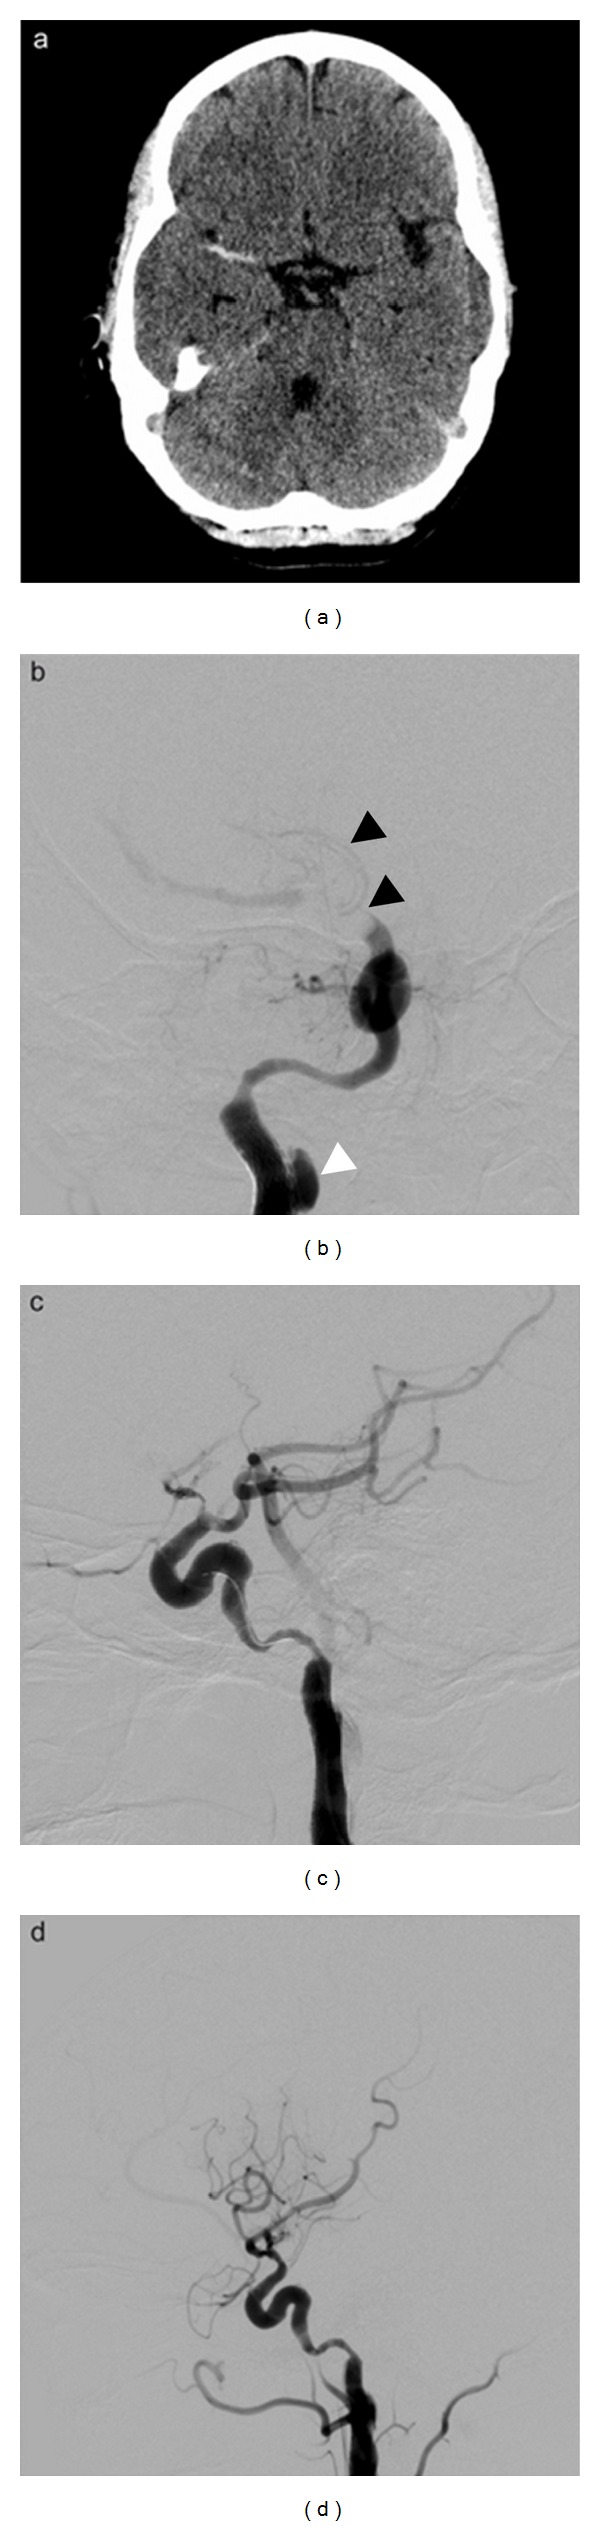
(a) Prethrombolysis CT showing hyperdense vessel sign in right MCA. (b) Angiogram after right ICA stenting and before Solitaire AB deployment showing pseudoaneurysm at the dissection site (white arrowhead) and thrombus within the ACA and M1 (black arrowheads). (c) Solitaire AB deployment with stent in situ. (d) Angiogram after clot retrieval from right MCA showing recanalization and reperfusion.
